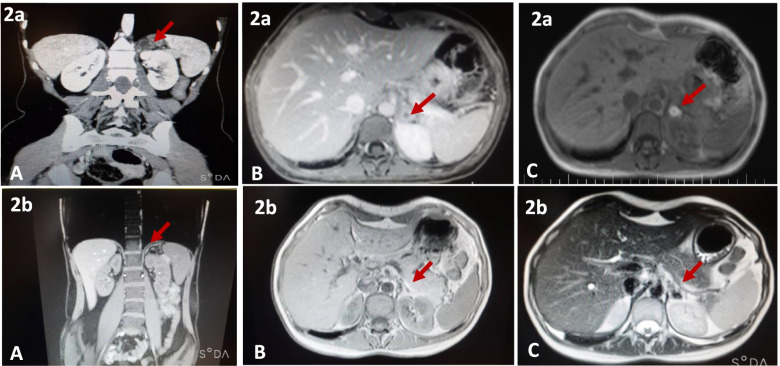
a. Abdominal magnetic resonance imaging (MRI) with gadolinium showed an oval shape well-encapsulated cystic mass with a thick and low signal intensity rim in the left adrenal gland(A: Coronal &B: axial view of hepatobiliary phase, C: hyperintense on T1 fat-saturated). The red arrows indicate adrenal hemorrhage. b. MRI with gadolinium showed near-complete resolution of left adrenal hematoma and residual hemosiderin is noted (A: Coronal & B: axial view of hepatobiliary phase, C: hyperintense on T1 fat-saturated)
